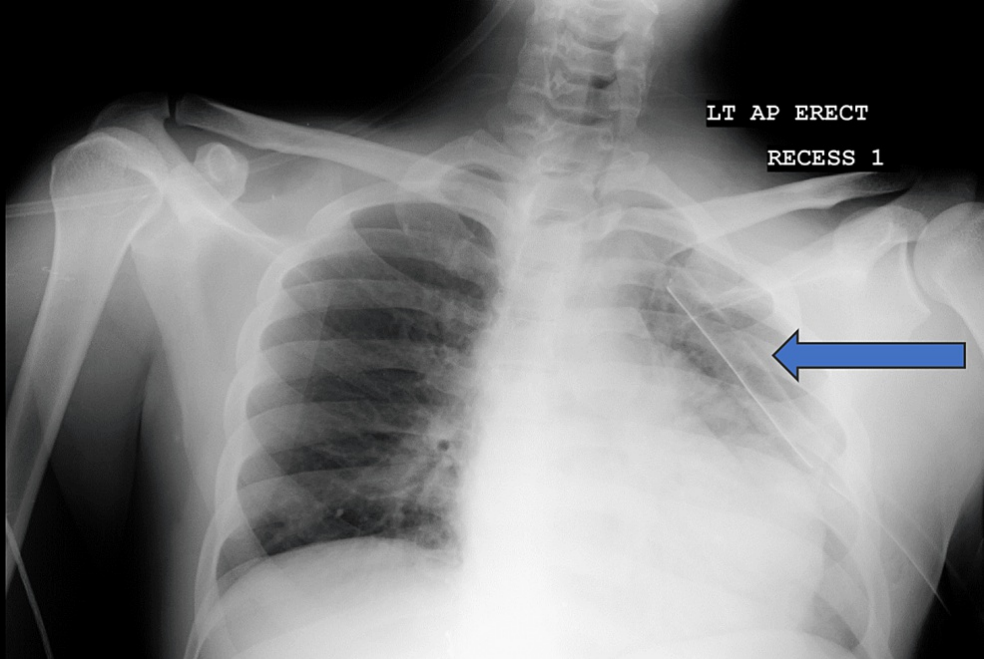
Pre-operative chest X-ray done in the emergency department after insertion of the intercostal chest tube into the left pleural space. The blue arrow indicates the intercostal chest tube.
